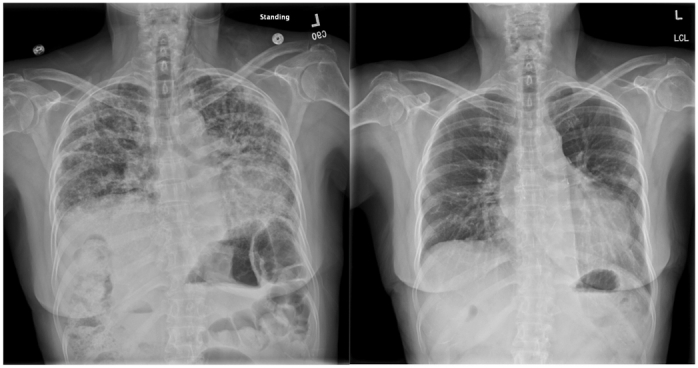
Chest radiographs from before and 9 months after lung transplant.
